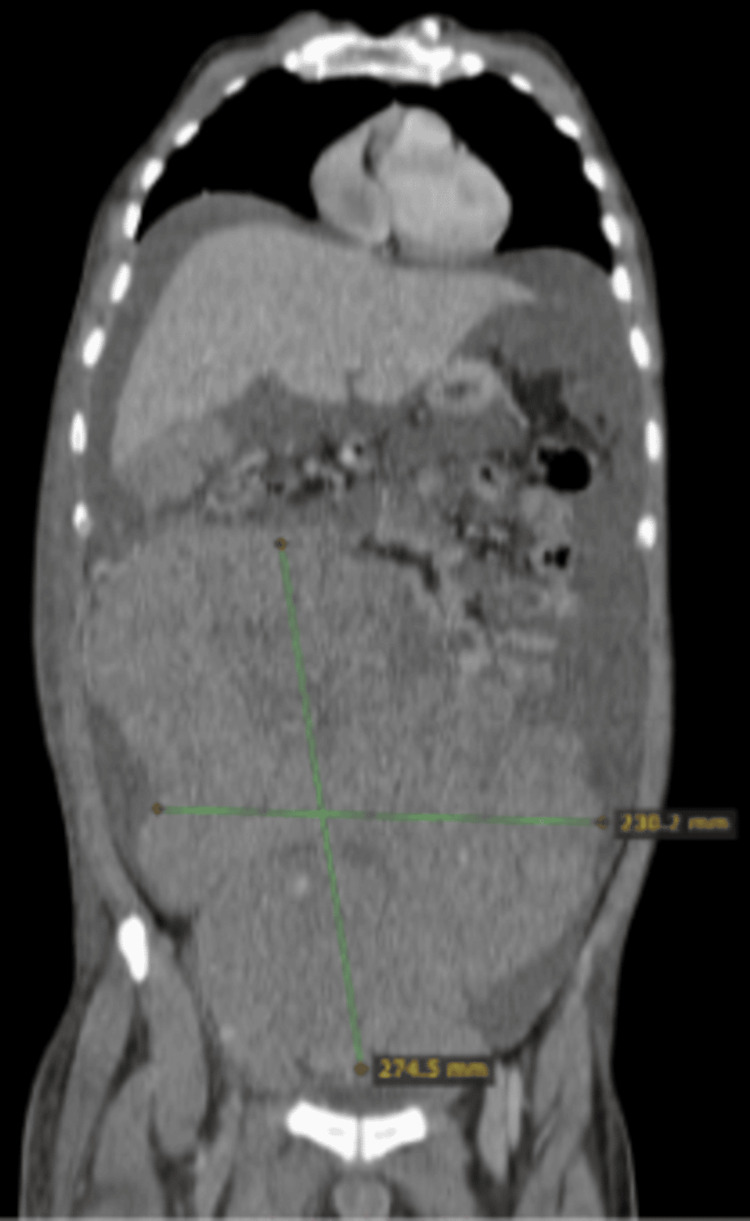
Coronal image of a CT scan showing around 23×17 cm mass in the pelvis CT: computed tomography
